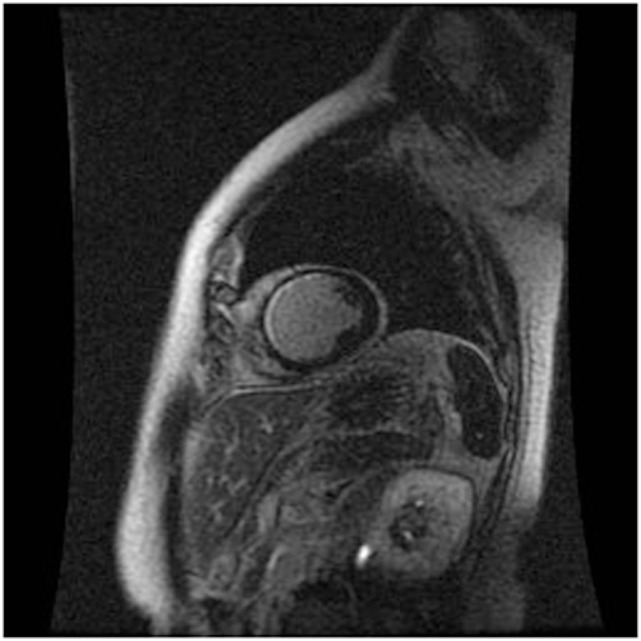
Cardiac CT: patient with high-grade left anterior descending artery stenosis (arrow).
